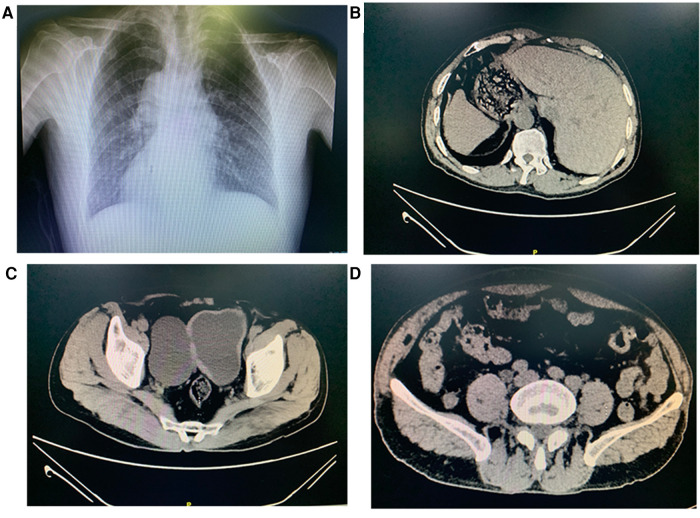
Chest radiographs show dextrocardia (A). Abnormal placement of stomach and liver (B). Computed tomography demonstrating a 78 × 50 mm diverticulum communicating with the bladder (C). Computed tomography demonstrating dilated hydronephrosis in the upper segment of the right-side ureteral (D).
